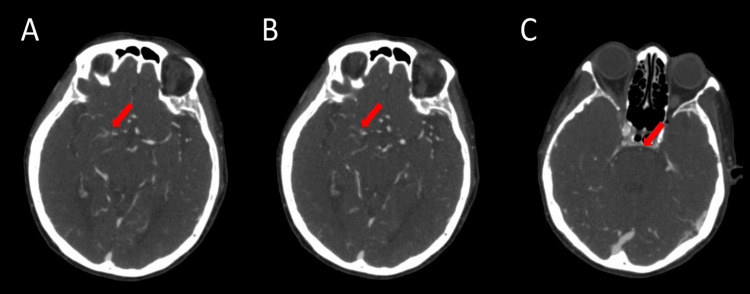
CT angiography of the head (A, B) Two consecutive axial slices showing focal occlusion of the right middle cerebral artery at the proximal M1 segment with prominent collateral flow into its peripheral branches. (C) Axial sequence showing stenosis of the basilar artery. CT: computed tomography. Red arrow indicates the pathology of interest.
